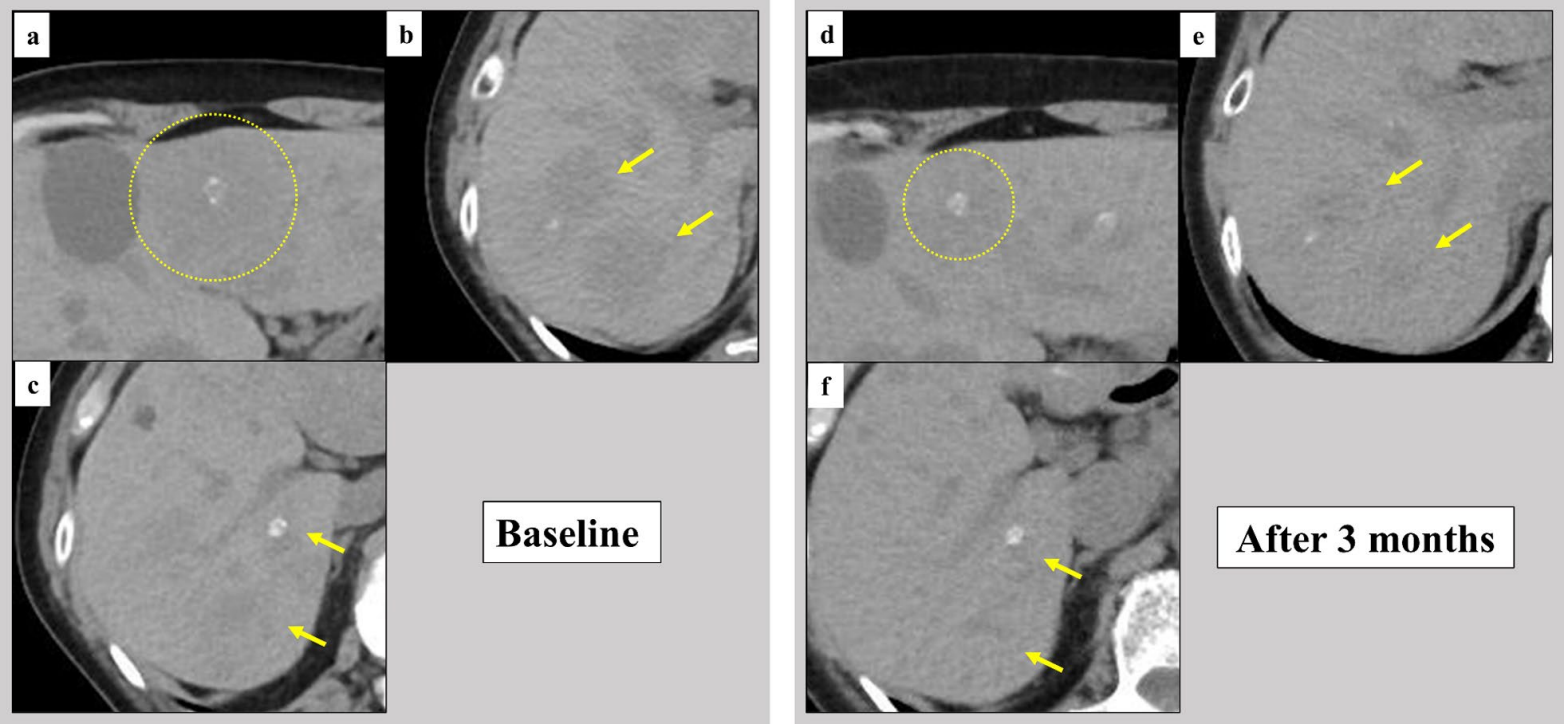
Baseline and 3 months after larotrectinib computed tomography images. The computed tomography before the initiation of larotrectinib showed multiple liver metastases with a maximum diameter of 48 mm (a–c). After 3 months of larotrectinib treatment, a 38% reduction in the tumor volume was achieved as the best clinical response (d–f)
